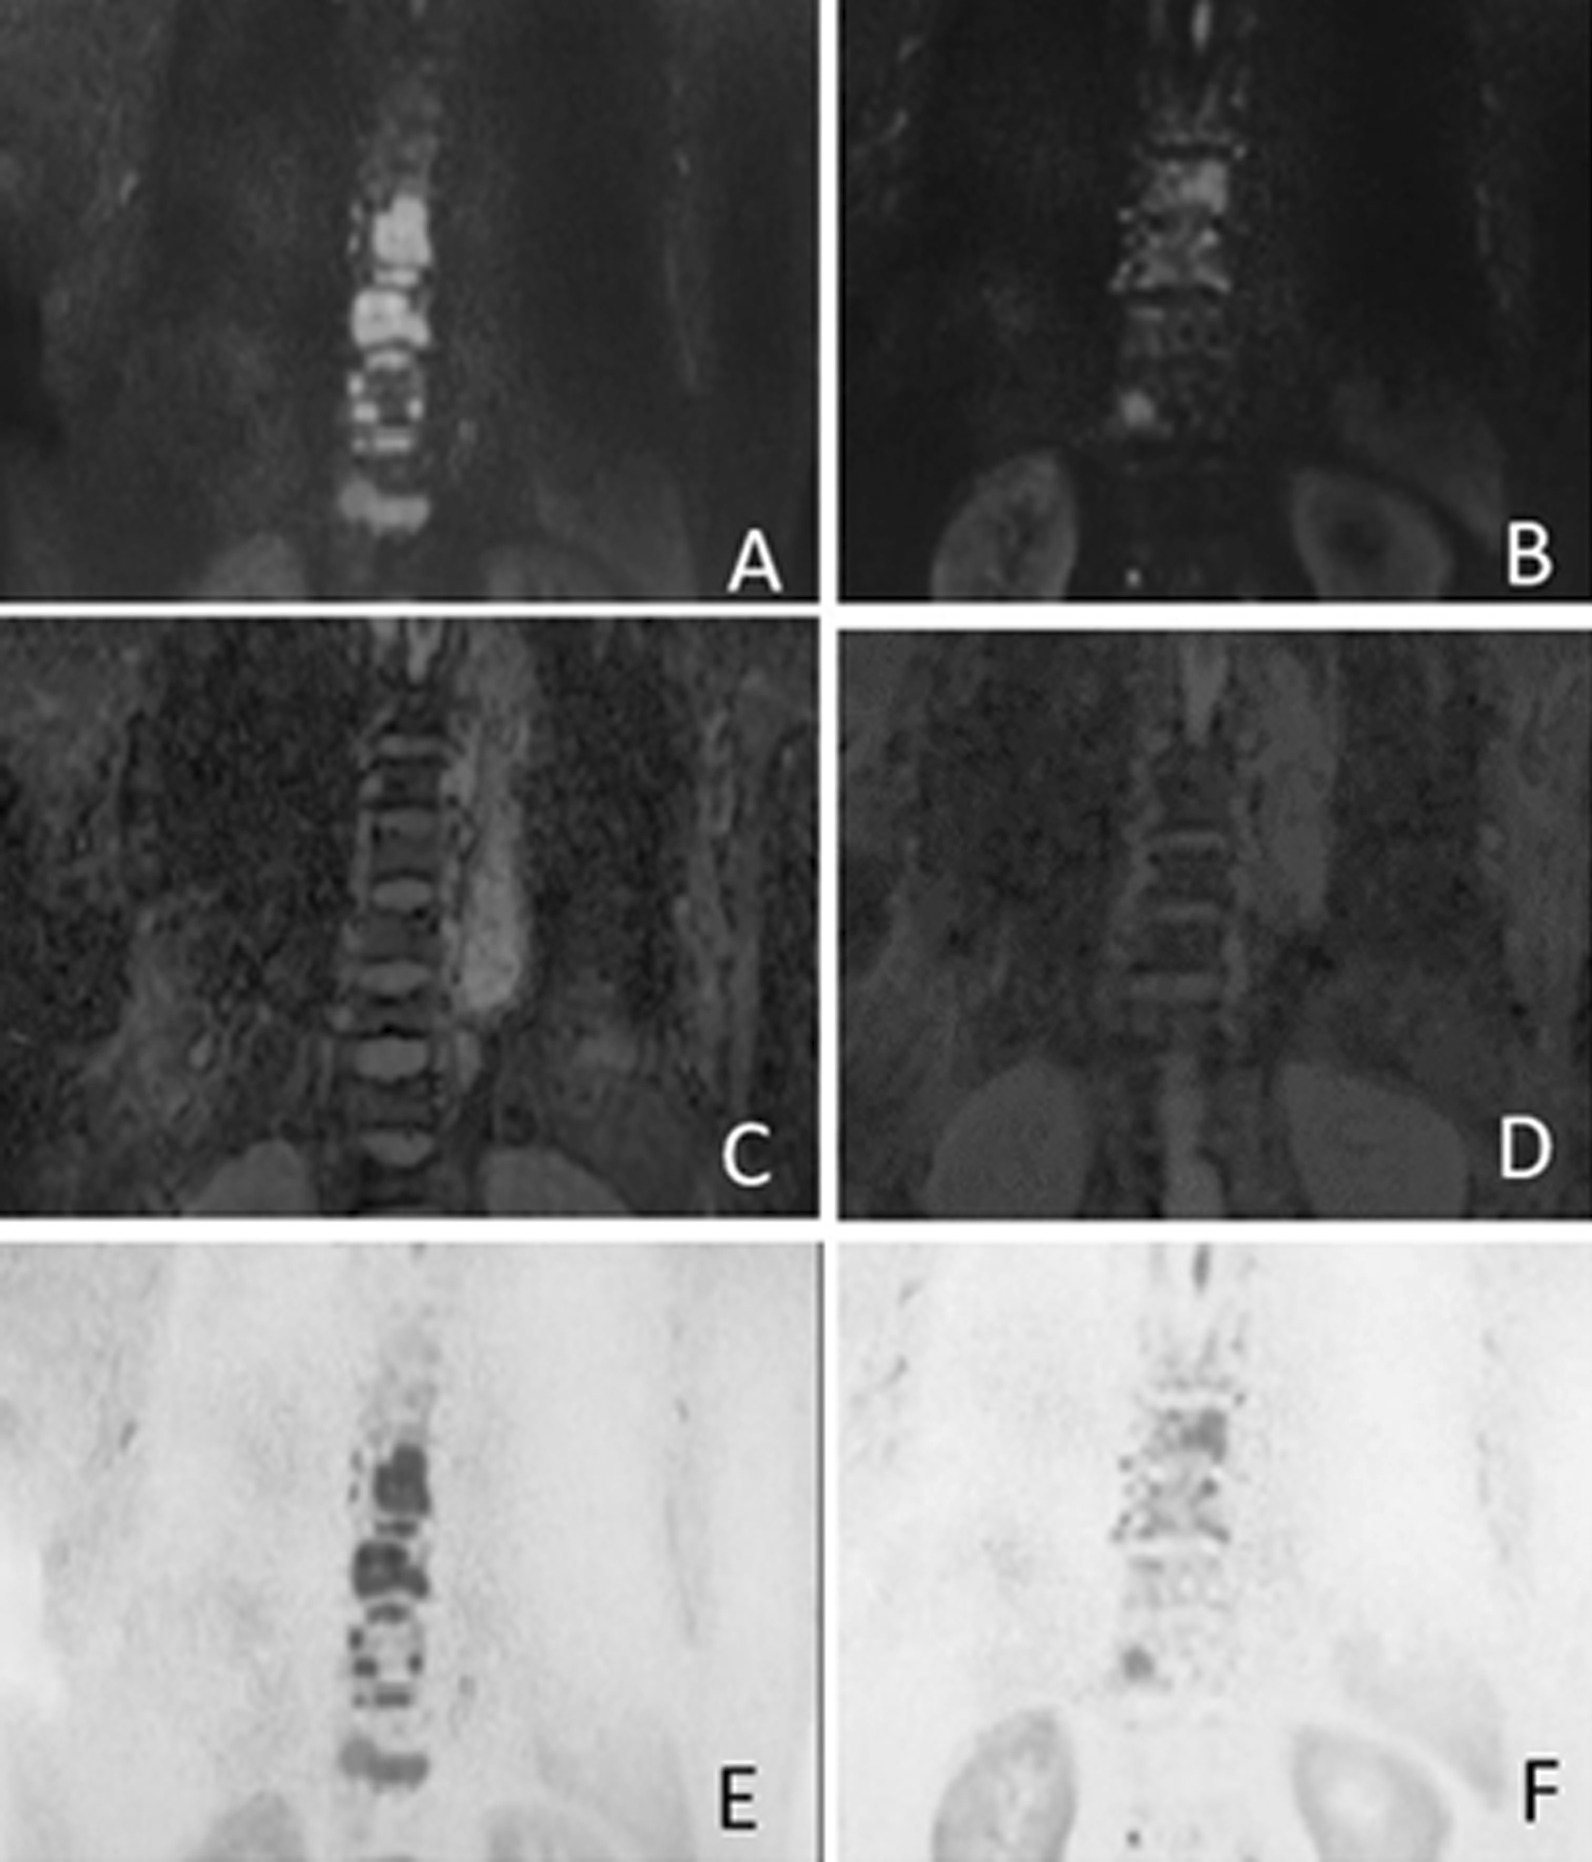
The patient underwent WB-DWI after the diagnosis of multiple myeloma. A (DWI b = 1000 s/mm^2^), C (ADC map) and E (inverted MIP image) show MM lesions of spine at baseline visit. B (DWI b = 1000 s/mm^2^), D (ADC map) and F (inverted MIP image) show MM lesions after 4-course chemotherapy. The ADC value of the lesion in the third lumbar vertebra increased from (0.698 × 10^–3^ mm^2^/s) to (0.796 × 10^–3^ mm^2^/s). In b = 1000 s/mm^2^ DWI images, the lesion area in B after treatment was significantly smaller than that in A before treatment. This contrast is more intuitive on the inverted image, which is shown in F after treatment and E before treatment
